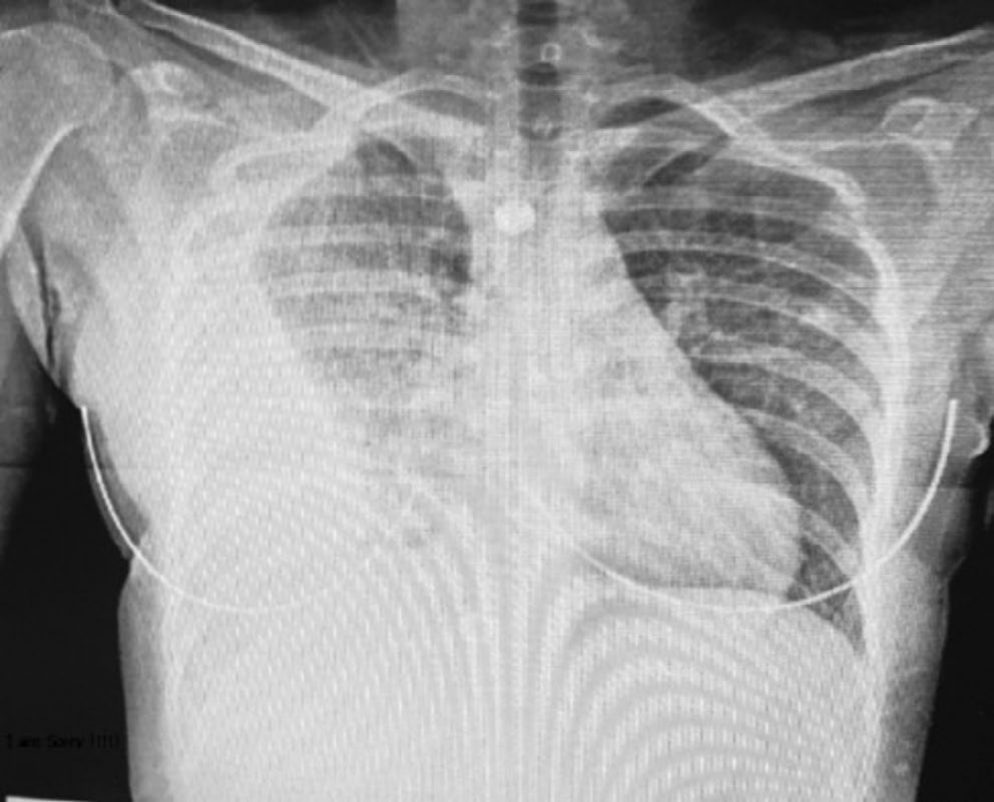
Preoperative posteroanterior chest radiograph showing complete right‐sided opacification with contralateral mediastinal shift.
